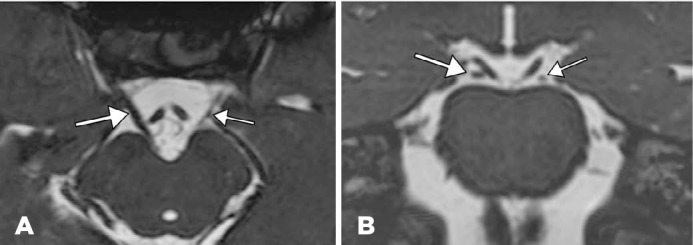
Magnetic resonance imaging (MRI) revealed hypoplasia of the third cranial
